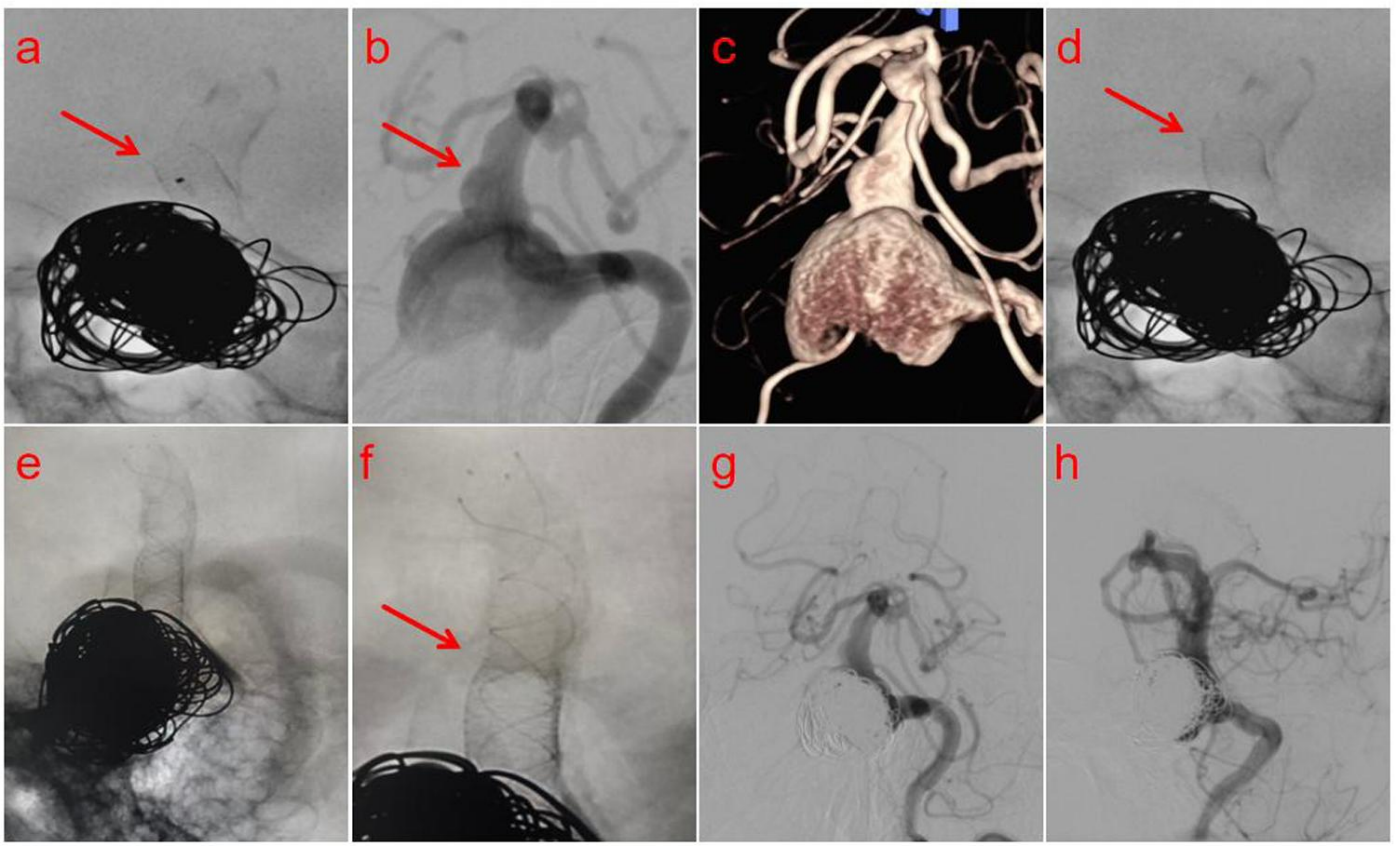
(a) Brain MRI revealed a round-like, space-occupying lesion in the left vertebral artery region with significant mass effect upon the adjacent brainstem. (b,c) Computed tomography angiography (CTA) of the brain demonstrated an aneurysm in the V4 segment of the left vertebral artery. (d,e) Digital subtraction angiography (DSA) confirmed a giant aneurysm of the left vertebral artery. (f) 3D vascular reconstruction revealed an aneurysm measuring 26.1 mm × 23.4 mm. (g) The first coil was delivered into the aneurysm sac and successfully formed the initial frame. (h) Ten coils were deployed into the aneurysm, resulting in complete sealing of the neck.
